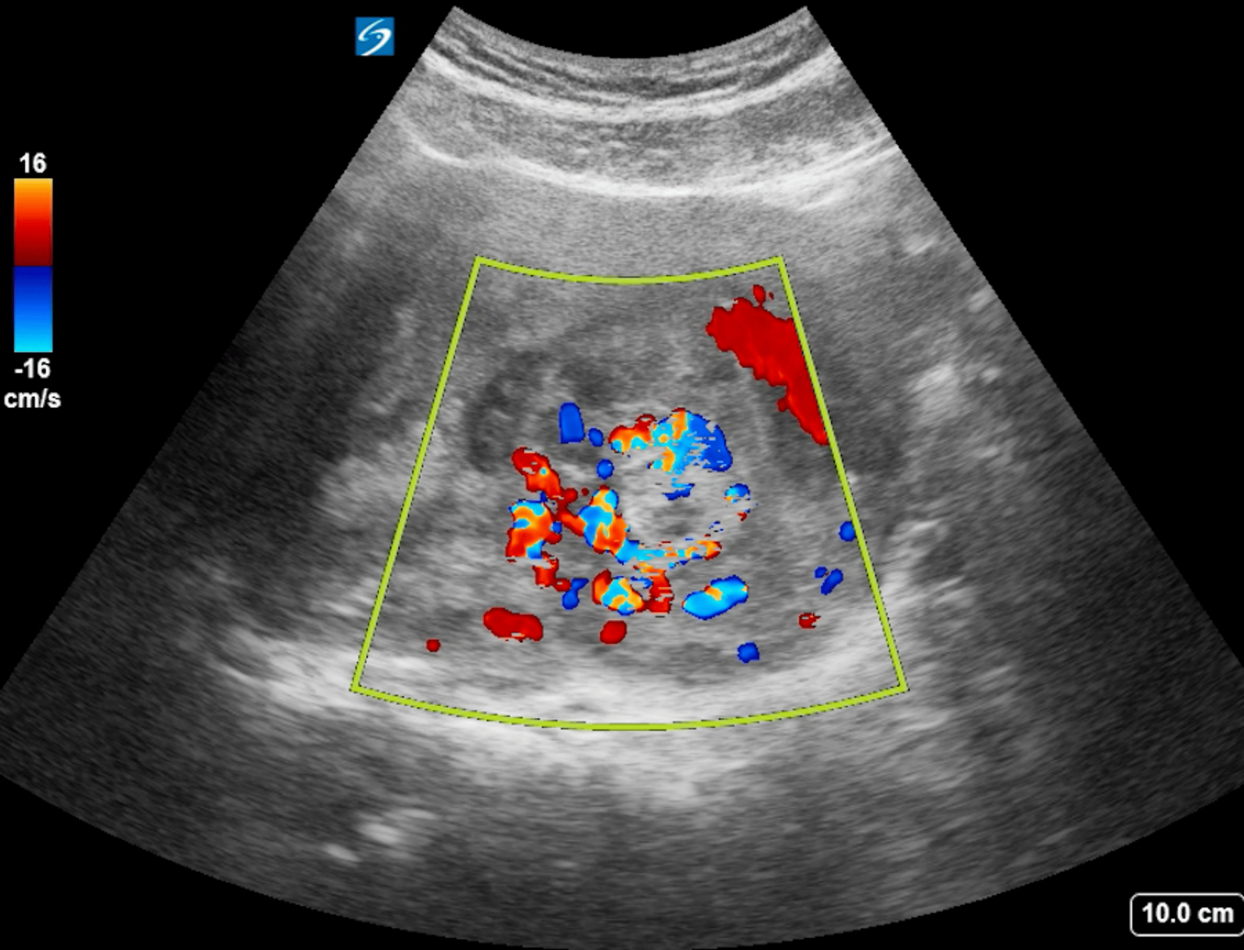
Transverse Uterus Color Doppler In this transverse view of the uterus, obtained via a transabdominal technique, color Doppler is applied to the thickened, echogenic endometrial material, displaying internal vascularity. This finding is highly specific for retained products of conception.
